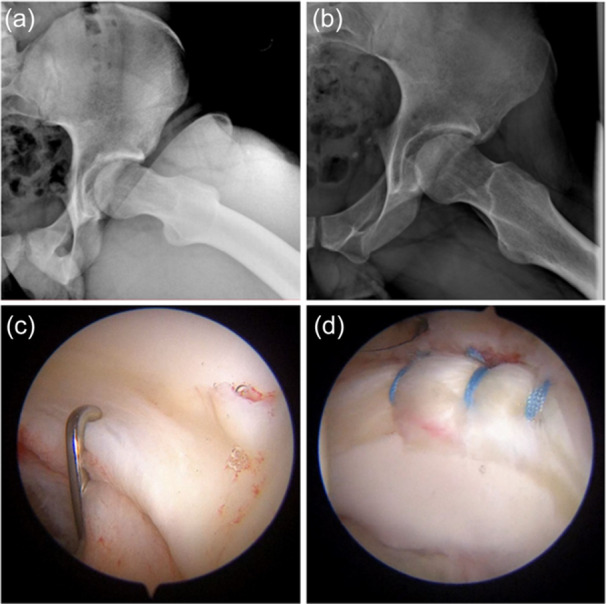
Representative radiographic and arthroscopic findings in a patient undergoing hip arthroscopy for femoroacetabular impingement. (a) Preoperative Dunn view showing cam‐type deformity. (b) Postoperative Dunn view after femoral osteoplasty. (c) Arthroscopic view of acetabular cartilage lesion. (d) Arthroscopic view following labral repair.
